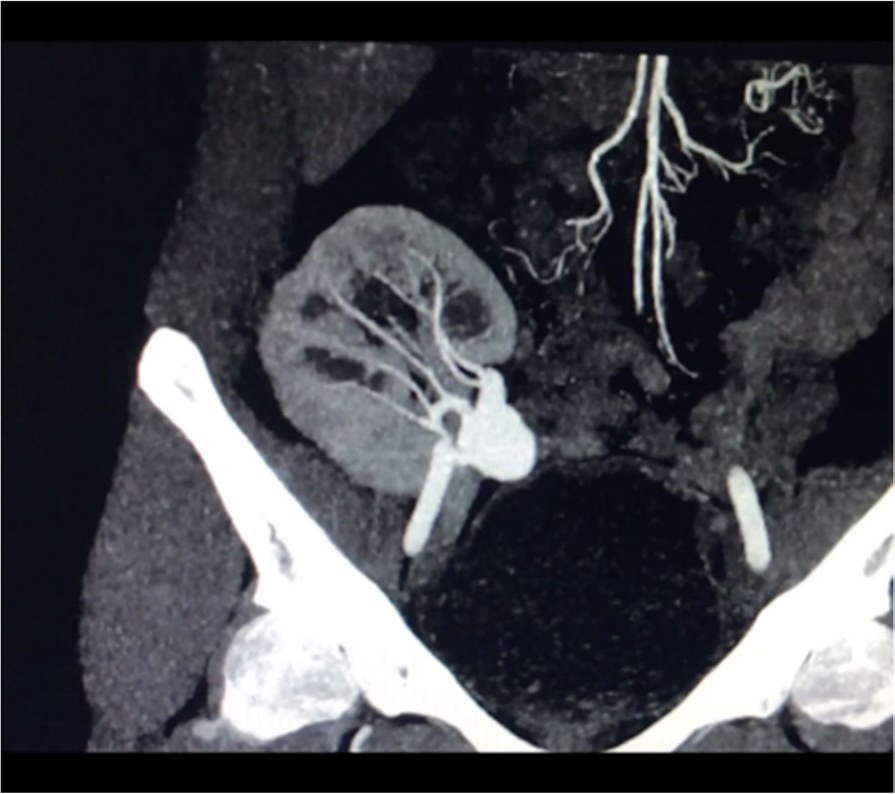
CT angiography showing large TRAA
